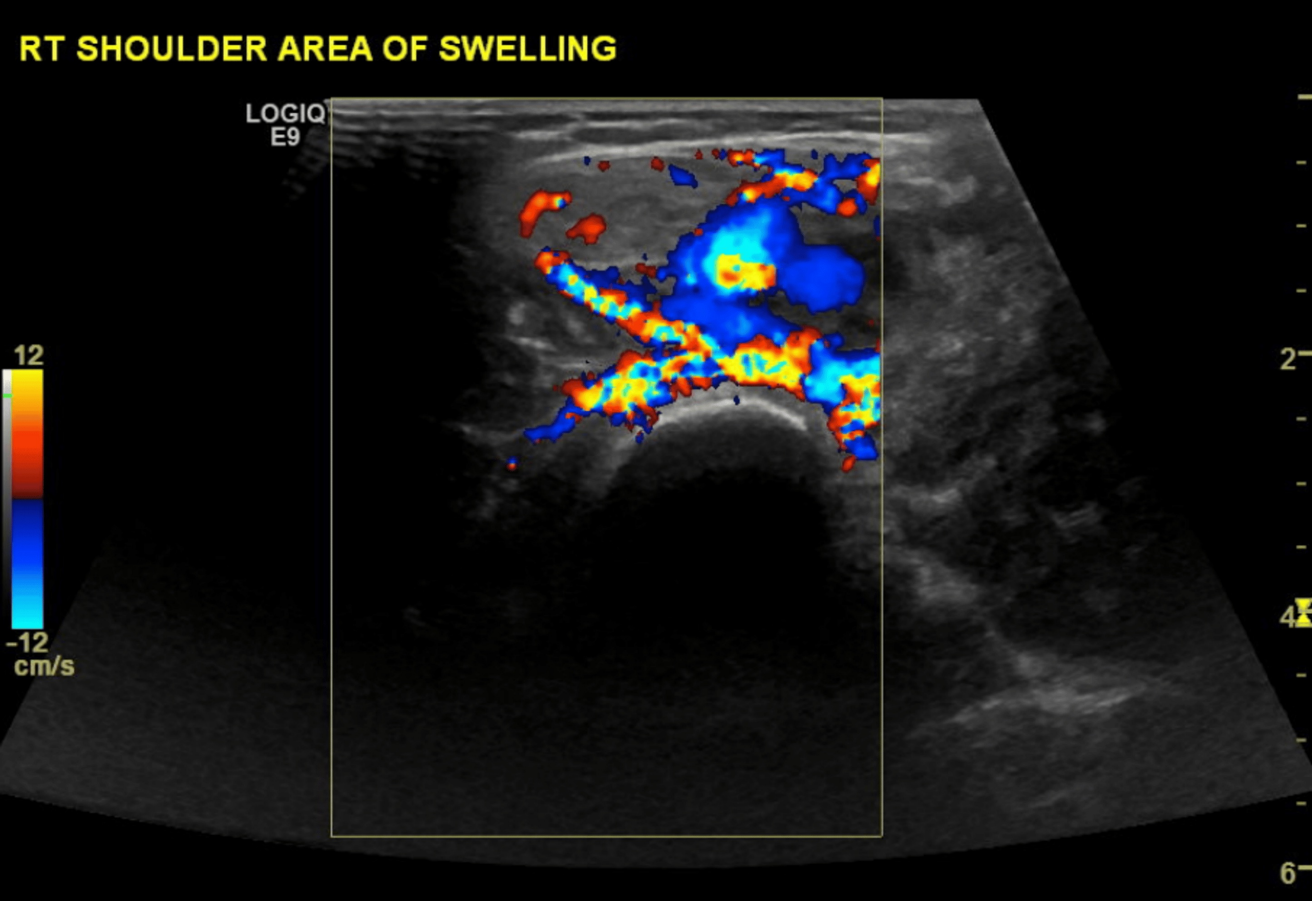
Ultrasound of right deltoid muscle High-flow arteriovenous malformation (AVM) within the right deltoid muscle, with no evidence of hematoma or fluid collection. Doppler ultrasound showed high diastolic venous flow and arterial flow consistent with an AVM.
